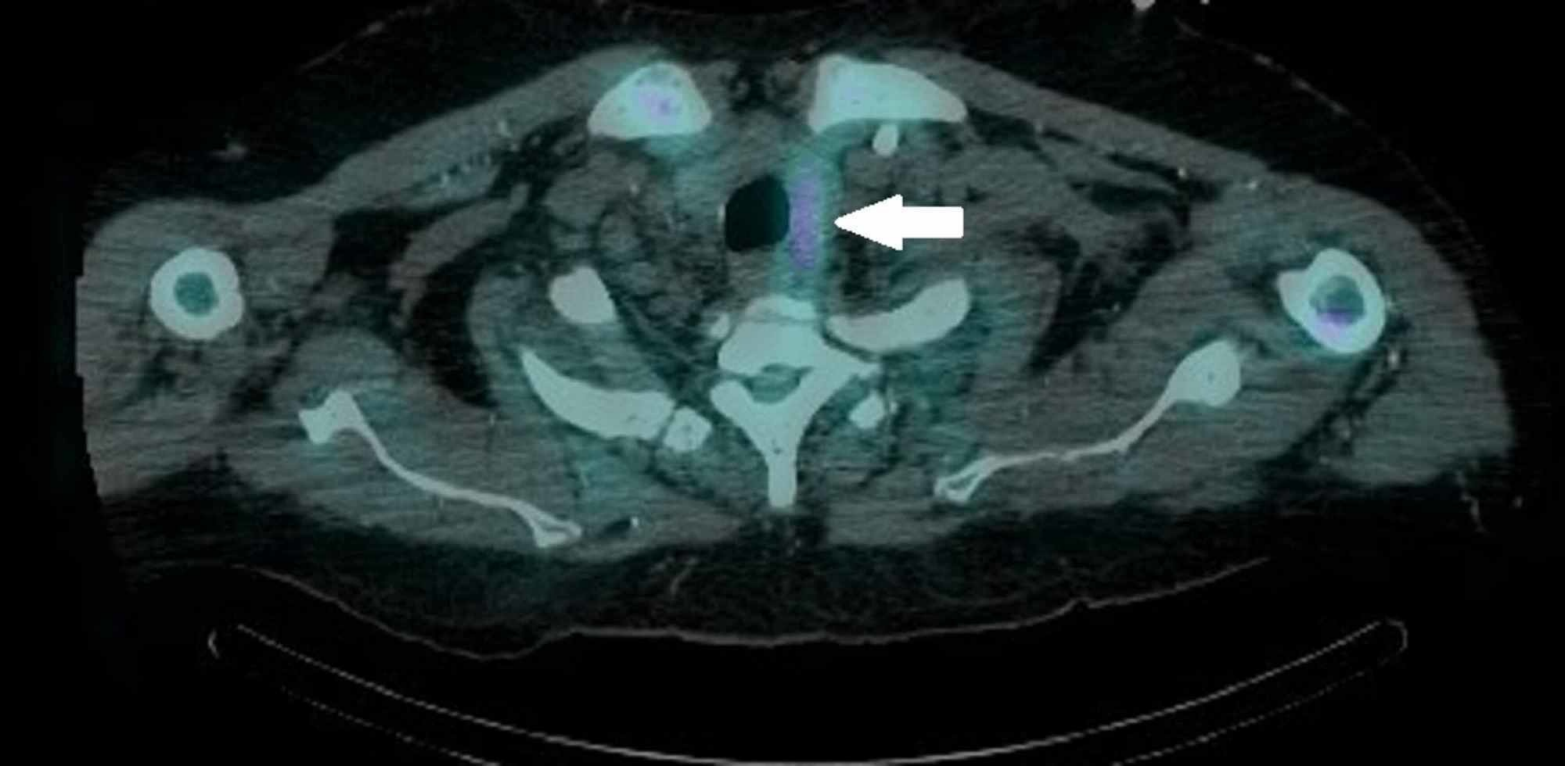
4D-CT scan of the lower neck demonstrates a 1.5 x 2 x 1.5 cm nodule with increased radiotracer uptake posterior to the lower pole of the left thyroid gland consistent with residual parathyroid tissue or parathyroid adenoma (thick white arrow).
